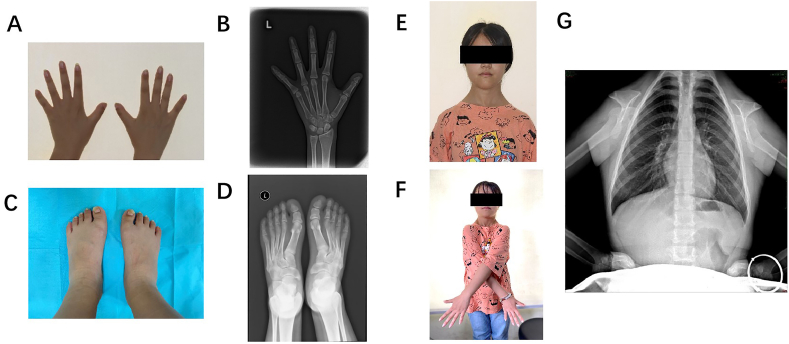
Clinical and radiographic findings of the proband's whole body. Photographing the patient's hand and radiographing the wrist bone (A and B). Photographs of the patient's feet and foot orthographic radiographs. The distal phalanges of the first toes were shortened, with irregular bony edges (C and D). Photographs show bilateral deformity and pseudarthrosis of clavicles (E and F). Plain X-ray of the chest shows bilateral deformity and pseudarthrosis of the clavicles (G).
